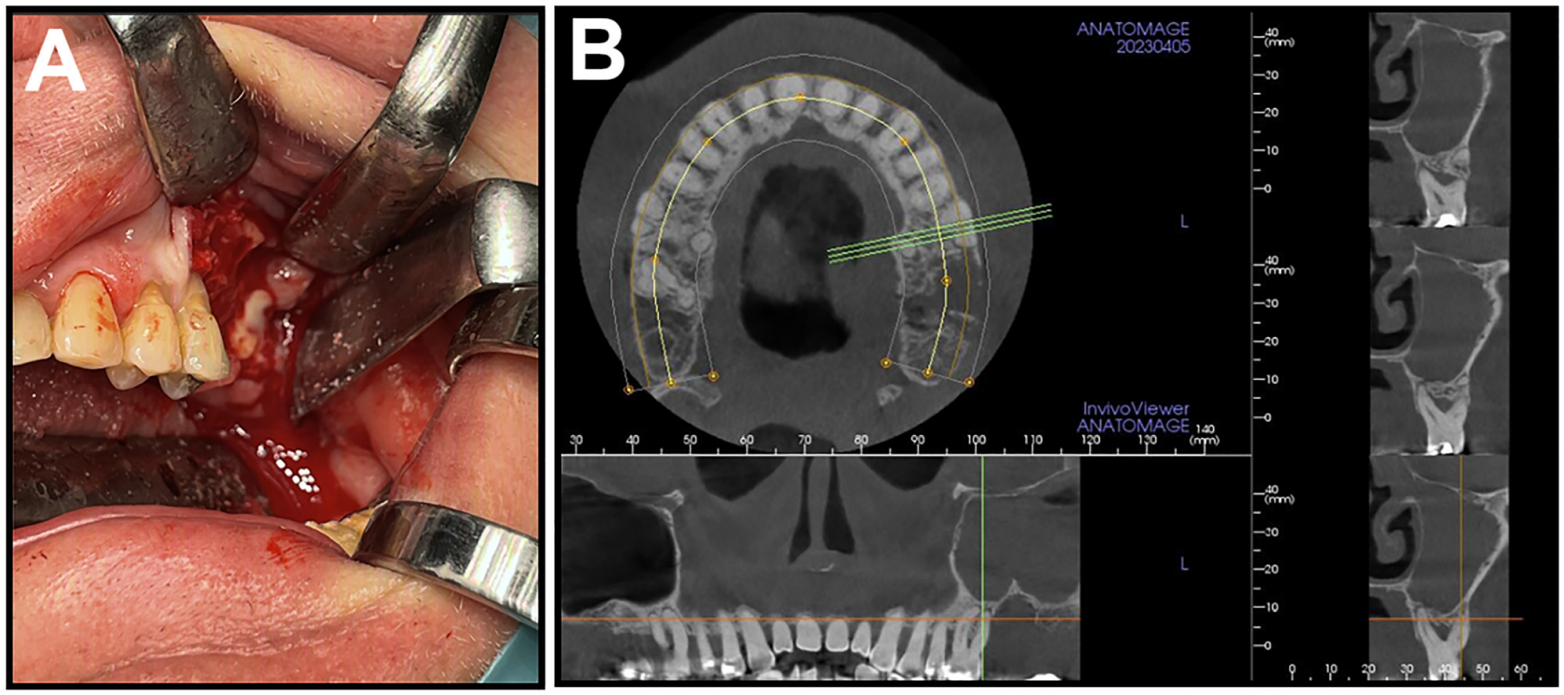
Clinical images corresponding to Case 2 A: Clinical presentation of the patient during surgery; B: Cone-beam CT (CBCT) image of patient before the surgical intervention
